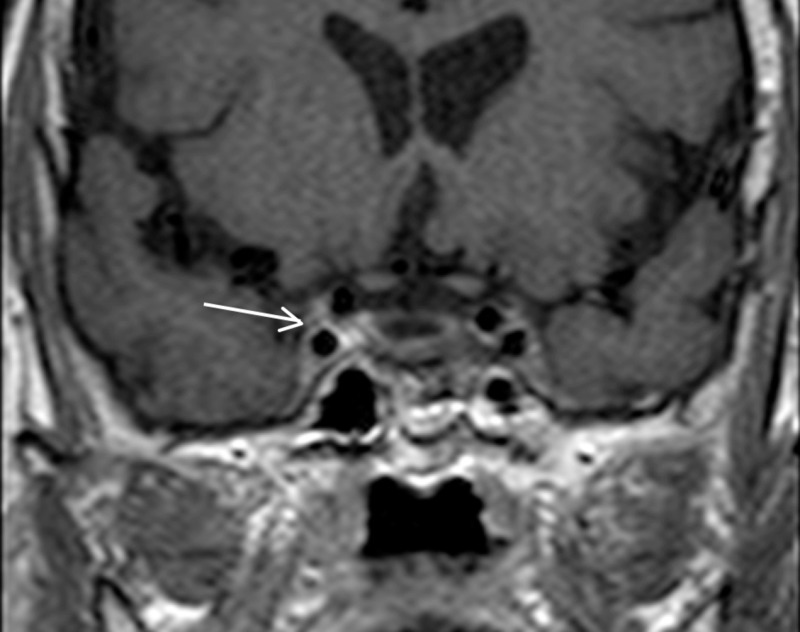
Cavernous sinus thrombophlebitis: coronal post-contrast T1WI (arrow) shows differential enhancement of the right cavernous sinus, suggestive of cavernous sinus thrombophlebitis T1WI - T1-weighted image
